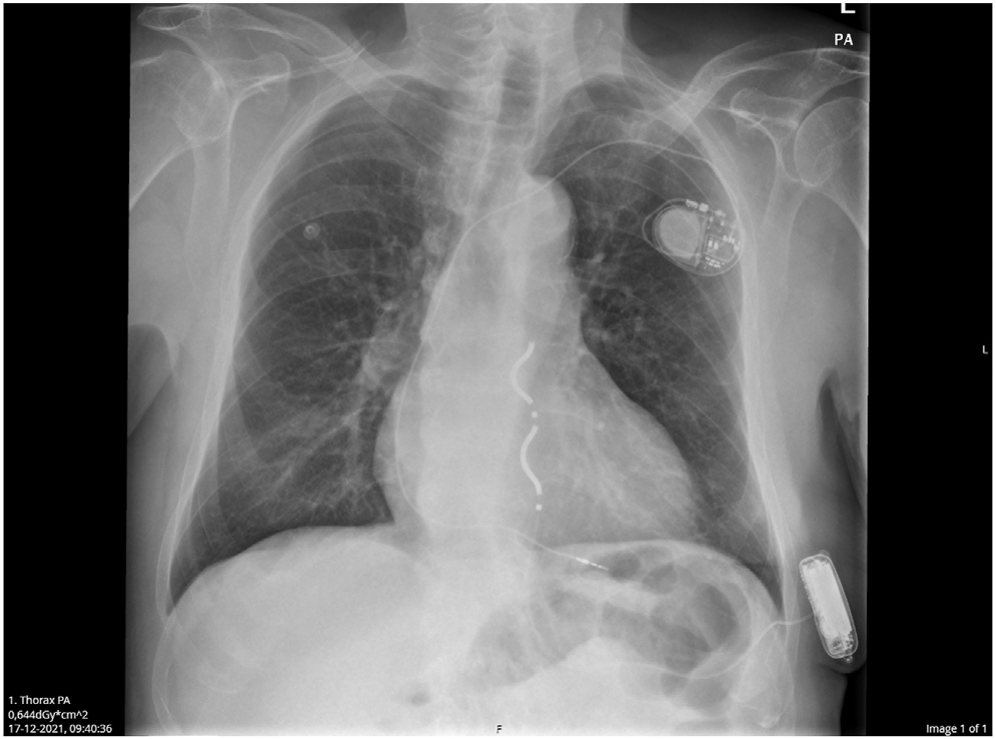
Radiography after device implant showing single-chamber pacemaker and extravascular implantable cardioverter-defibrillator.
